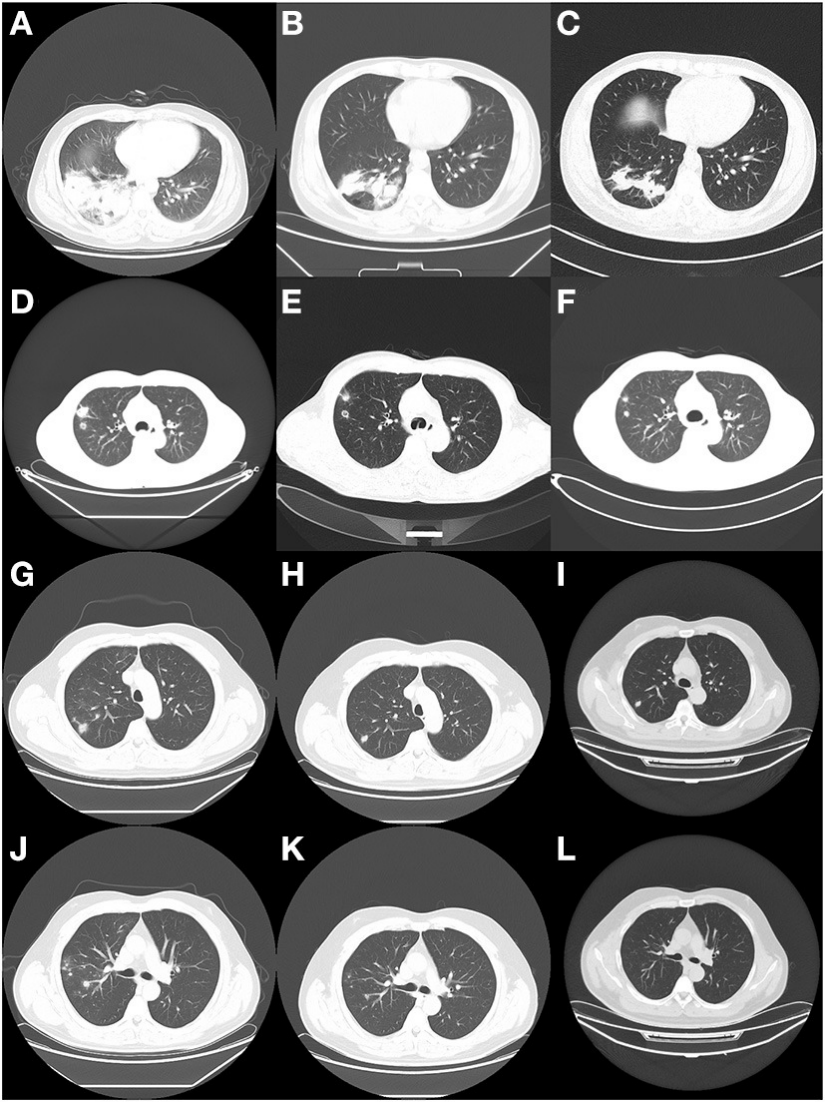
CT findings of the three patients at different periods. (A) The enhanced CT scan of patient 1 on the second day of admission showed a right lower lung lesion. (B) 1 month after discharge, CT reexamination of patient 1 showed a reduction of the lesion. (C) 3 months after discharge, CT reexamination of patient 1 showed a continuous reduction of the lesion. (D) CT scan of patient 2 on admission showed lung lesions. (E) 2 months after discharge, CT reexamination of patient 2 showed a reduction of the lesion. (F) 4 months after discharge, CT reexamination of patient 2 showed a smaller lesion than the previous one. (G,J) CT scan of patient 3 at the hospital showed lung lesions. (H,K) 6 months later, the patient was reexamined by CT. (I,L) 9 months later, the patient was reexamined by CT.
